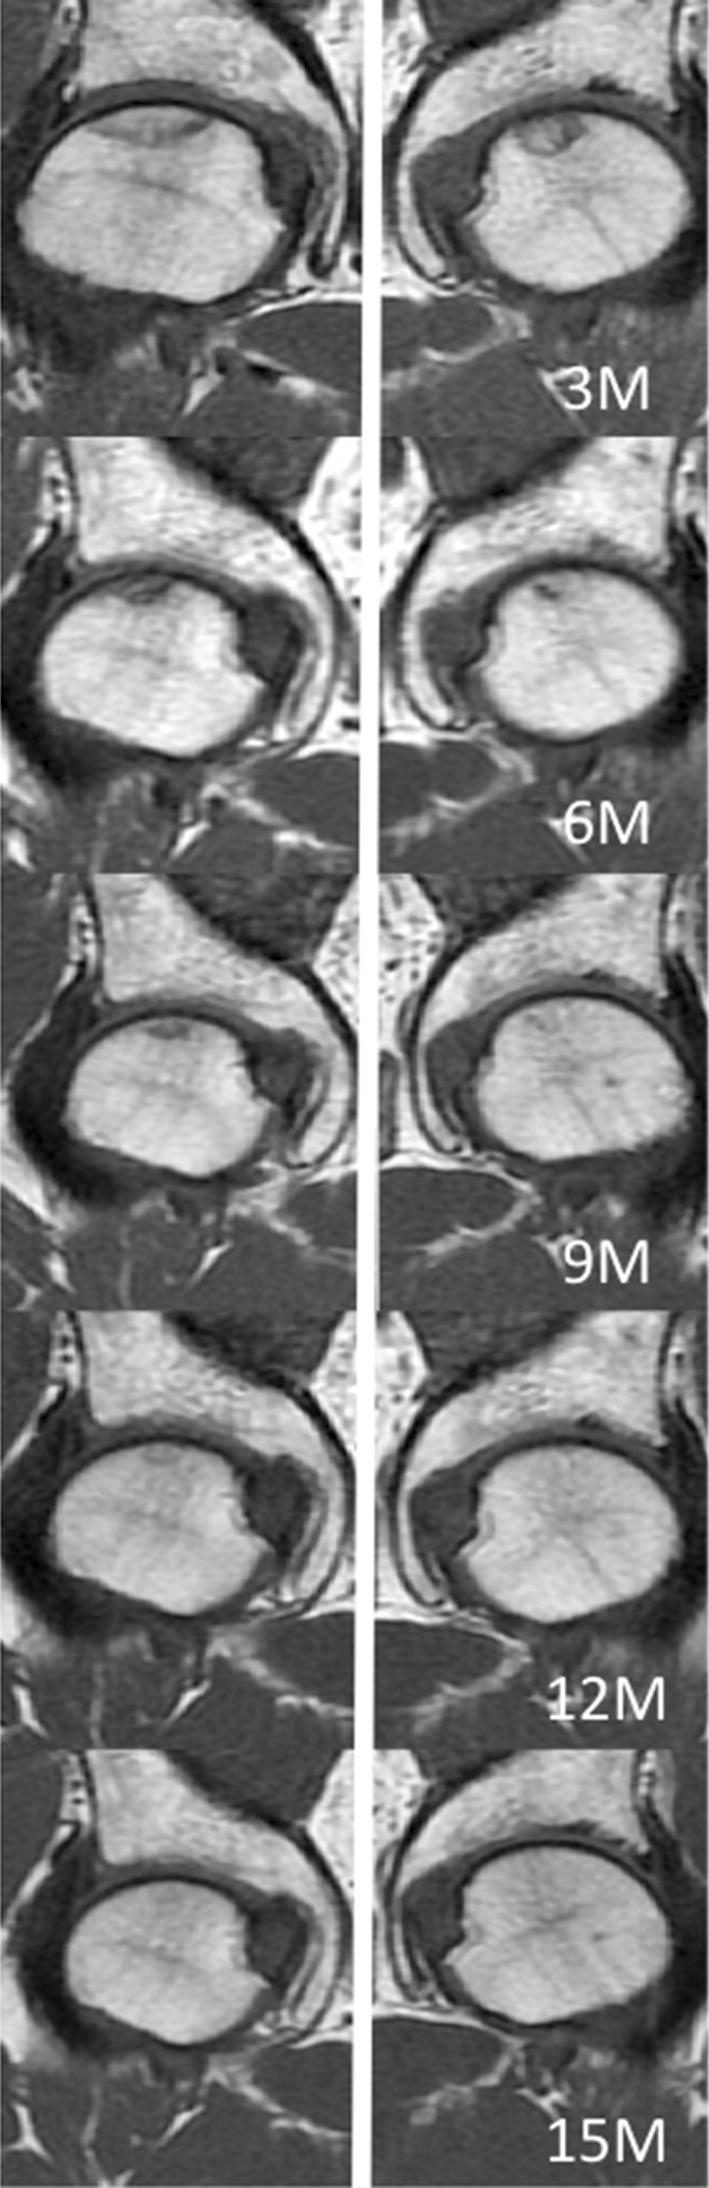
T1-weighted images of bilateral coronal MRI in an asymptomatic 57-year-old man. ONFH was detected at 3 months after corticosteroid treatment (Type C1 lesion in the right femoral head and Type B lesion in the left side). Six months after treatment, the ONFH lesion had decreased in size. The left femoral head ONFH lesion disappeared at 12 months after corticosteroid treatment and the right femoral head ONFH lesion disappeared at 15 months after corticosteroid treatment
